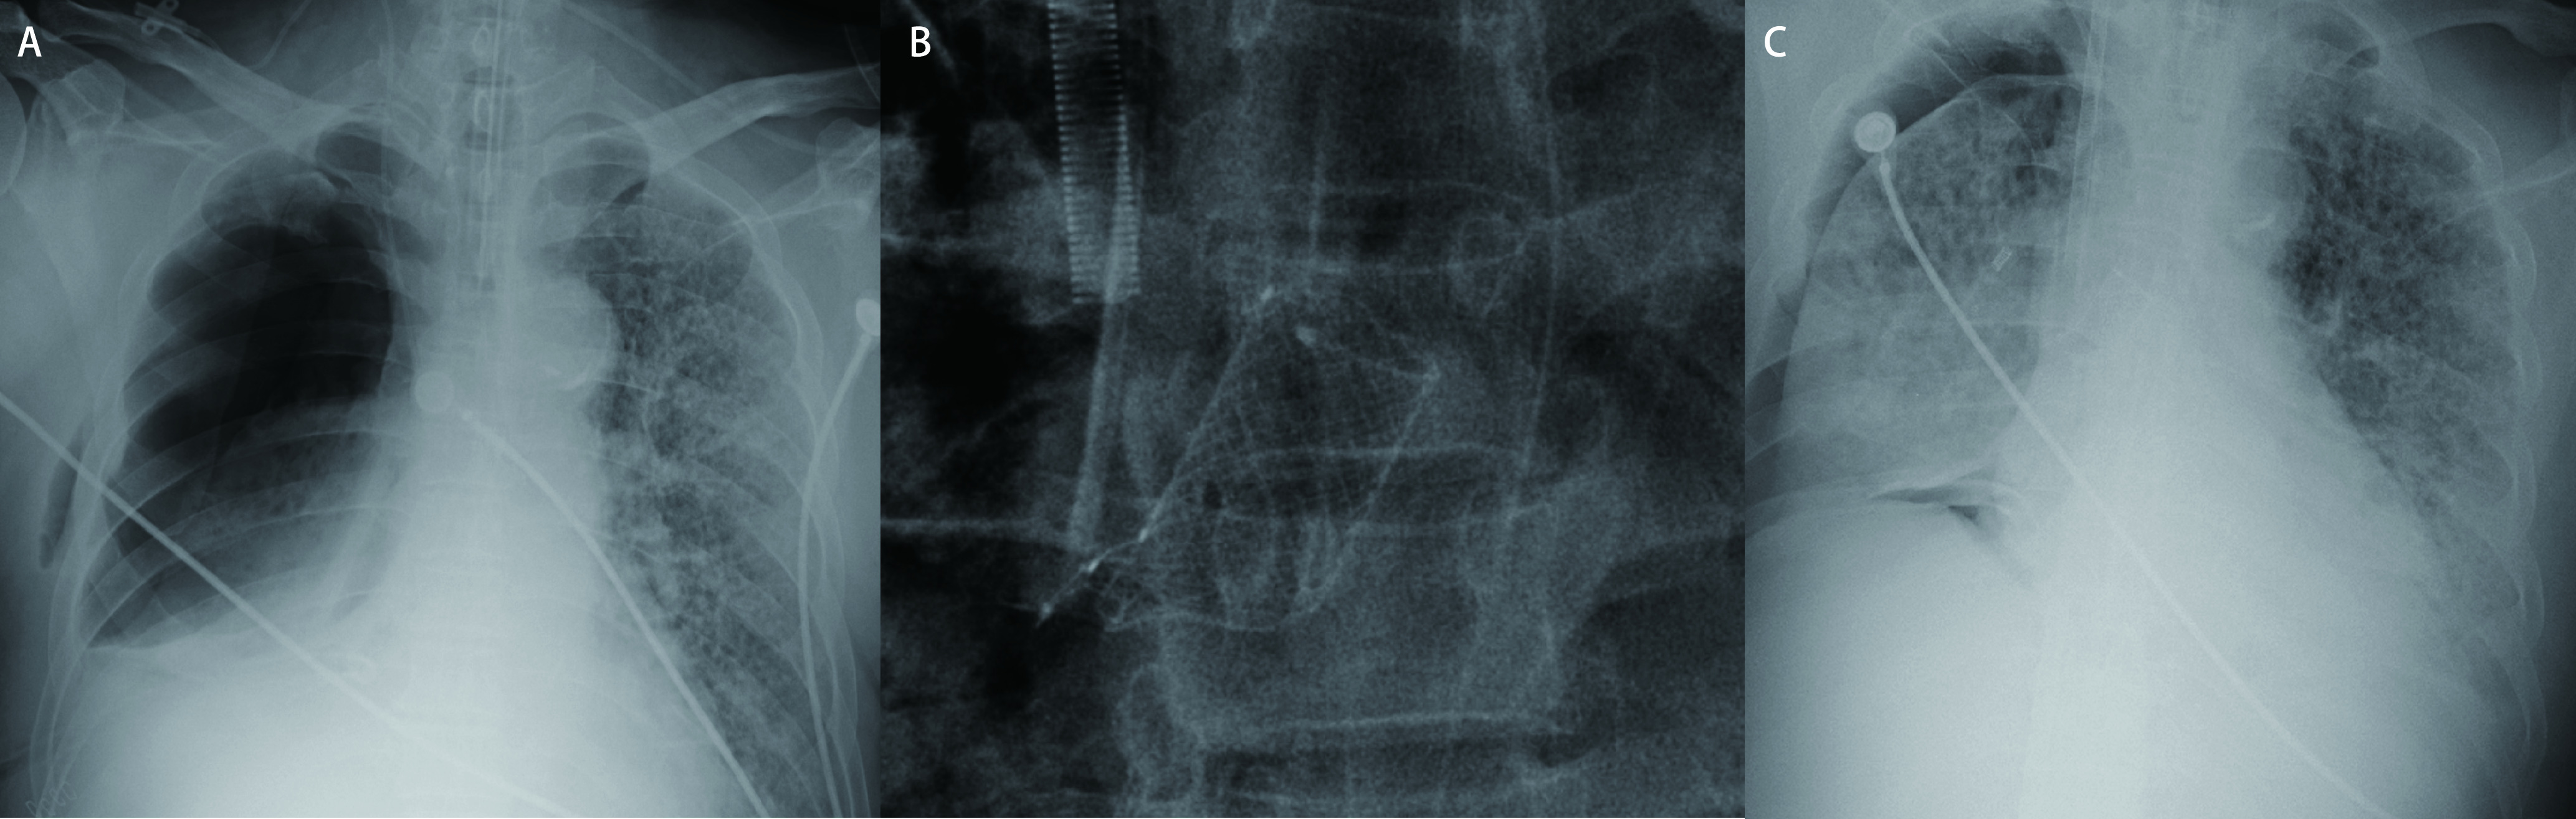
支架置入前后患者胸片情况。A：支气管残端瘘发生之后，右全肺不张; B：支架置入术后第1天，胸片上支架所在位置; C：支架封堵残端瘘口之后，右肺逐渐复张。 Chest X-ray before and after stent implantation. A: Right lung atelectasis after the bronchial stump fistula occurred; B: Location of the stent on chest X-ray on the first day after stent implantation; C: The right lung gradually re-expanded after the stent blocked the stump fistula.
